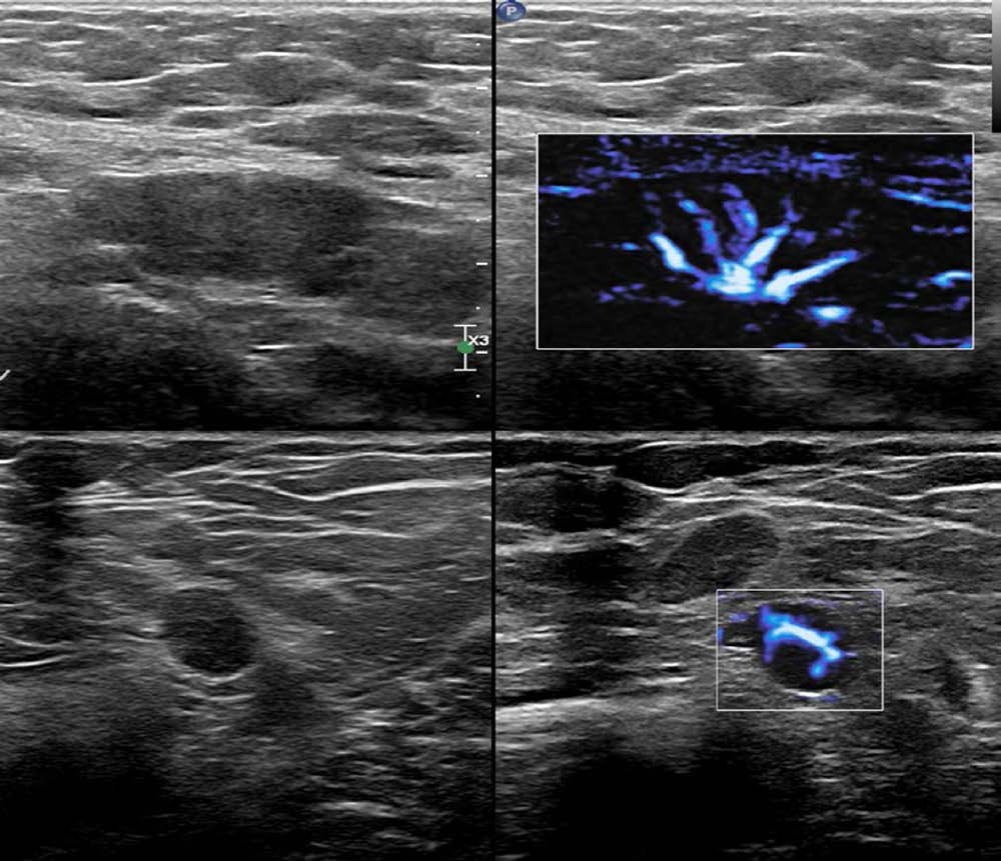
Transverse gray-scale ultrasonography (top left), showing axillary lymph nodes that were round and without hilums. Color Doppler ultrasonography (right), showing abundant hilar vascularity (top right). Bilateral inguinal lymph nodes had similar morphological and structural changes (bottom left and right).
